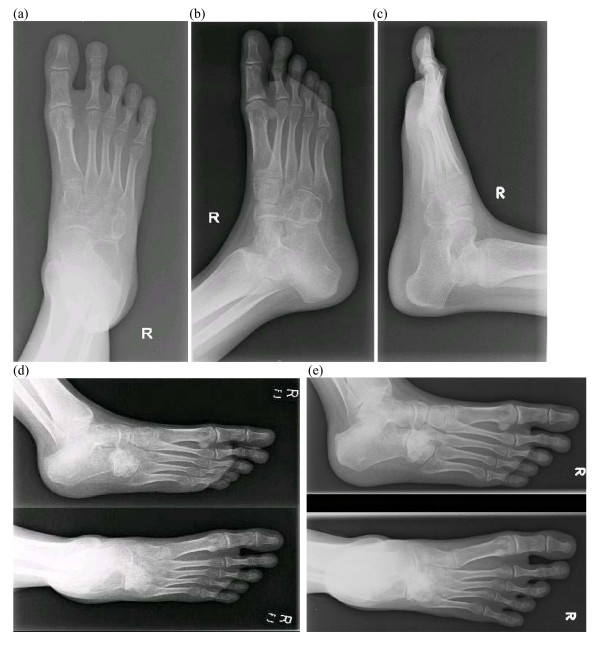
Conventional radiographs showed a lytic lucent area within the right cuboid bone. Septations were seen, but the cortex was intact. No fractures or dislocation of the cuboid was noted.
